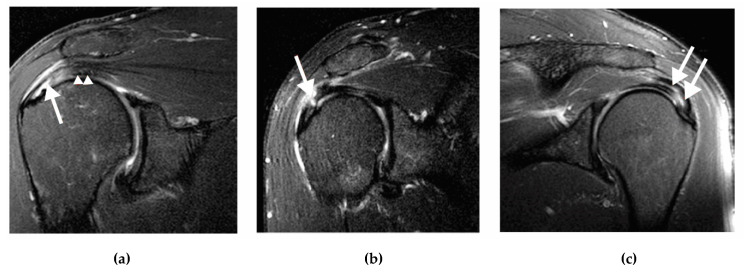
Coronal magnetic resonance imaging (MRIs) showing the three types of partial thickness of ST tears. (a) articular insertional tear (arrow), ST (arrow head); (b) bursal tear (arrow); (c) intratendinous tear (arrows). MRI, magnetic resonance imaging; ST, supraspinatus tendon.
